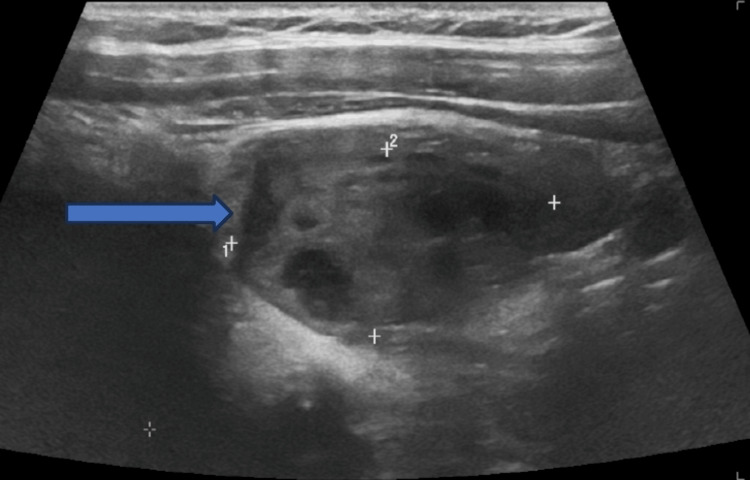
Ultrasound of the neck shows parathyroid adenoma as shown by the pointed arrow
